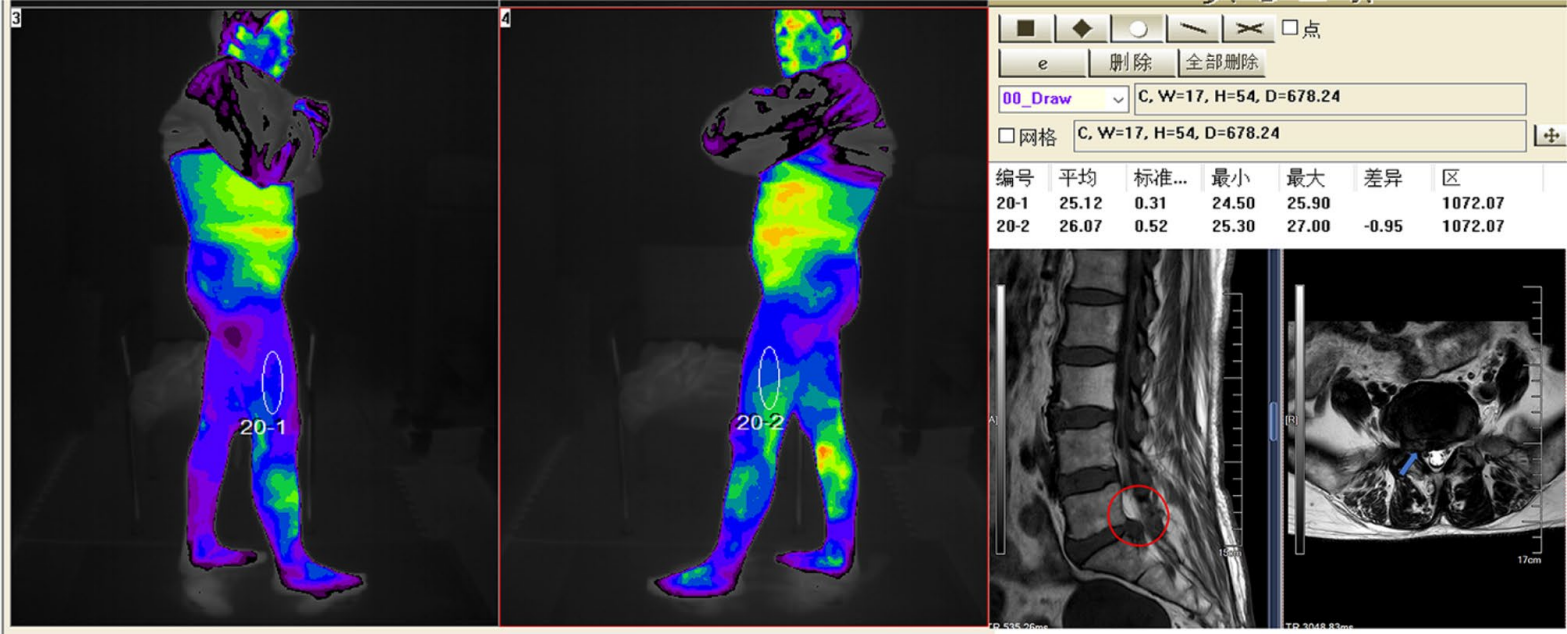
A 66-year-old female patient experienced radiating pain in her right lower limb for 20 days, predominantly in the outer thigh area. The IT examination showed a lower surface temperature in the affected area with a temperature difference of 0.95℃, compared to the unaffected side. A subsequent lumbar MRI revealed a lumbar disk herniation at the L5/S1 level on the right side, leading to nerve root compression. A percutaneous lumbar endoscopic discectomy was performed to address the issue, and during the surgery, the S1 nerve root was found to be closely associated with the intervertebral disc, exhibiting signs of ischemia and poor mobility. The successful decompression resulted in significant alleviation of the patient’s lower limb pain
